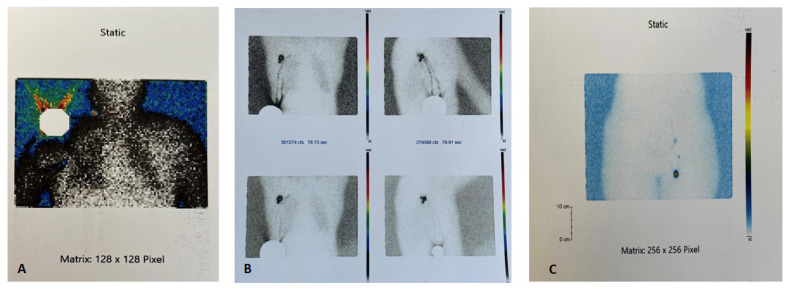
Lymphoscintigraphy aspect—Dr. Leventer Centre Collection. (A). Radiotracer capture on melanoma site (right shoulder). (B). Axillary sentinel lymph node. (C). Femoral sentinel lymph node.
